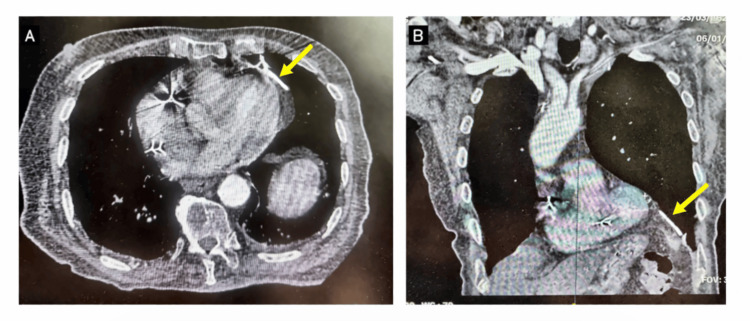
Computed tomography demonstrating pacemaker lead perforation with likely septal/paraseptal course, left ventricular free-wall exit, and extracardiac extension (A) Axial contrast-enhanced chest CT showing the pacemaker ventricular lead beyond the expected intracardiac course, with perforation through the left ventricular free wall and extension toward the left hemithorax. (B) Coronal CT reconstruction further demonstrates extracardiac extension of the lead toward the left pleural space and adjacent lung parenchyma. Although the original chest radiographs and additional lung-window CT reconstructions were unavailable for submission, the outside radiologic evaluation described a small associated left pneumothorax and focal pulmonary hematoma.
